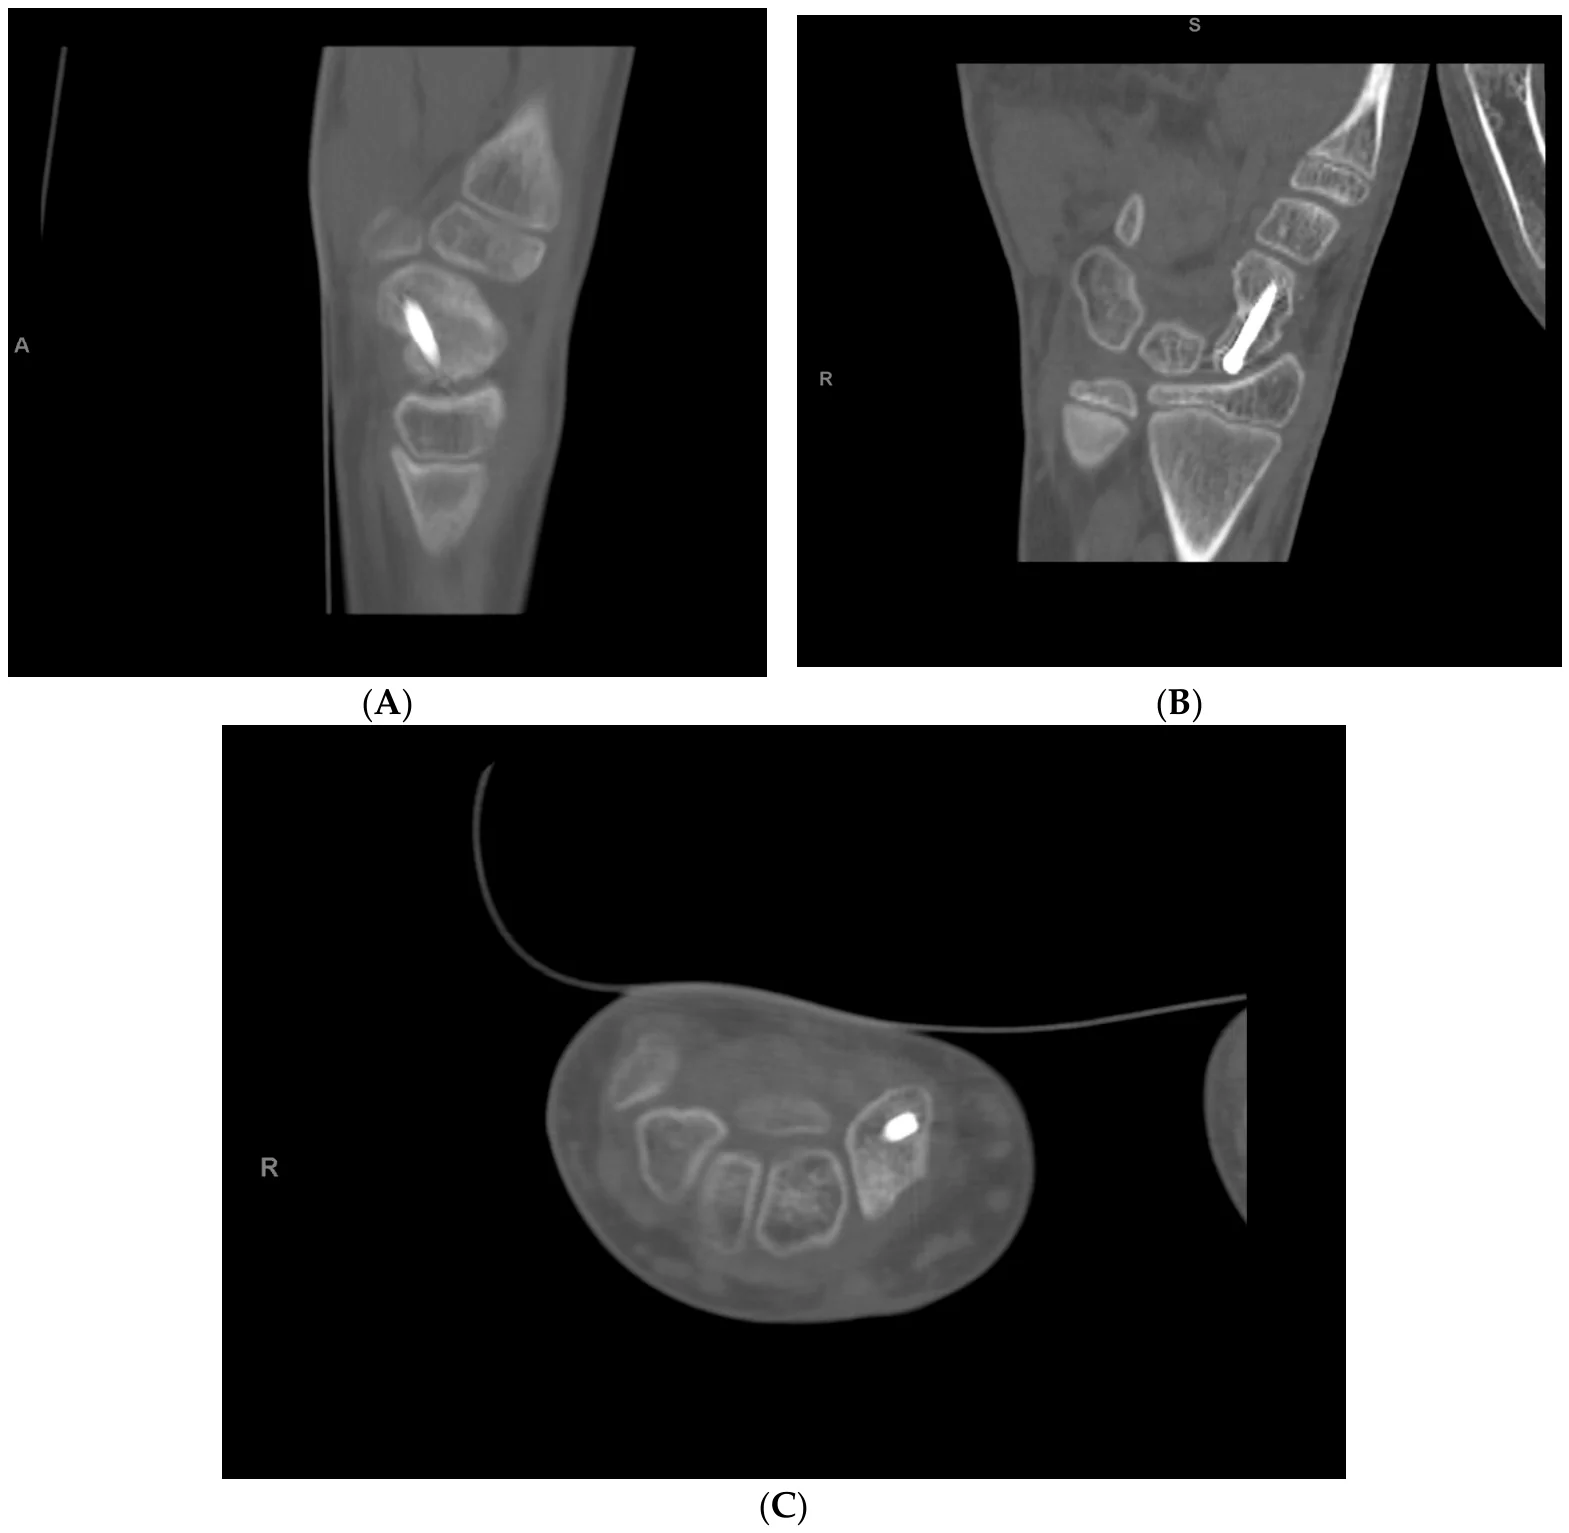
Six-month CT confirmation of union. (A) Sagittal, (B) coronal, and (C) axial CT images demonstrating bridging trabeculae across the former inferior-pole nonunion, confirming complete osseous union with maintained scaphoid alignment and stable screw position.
